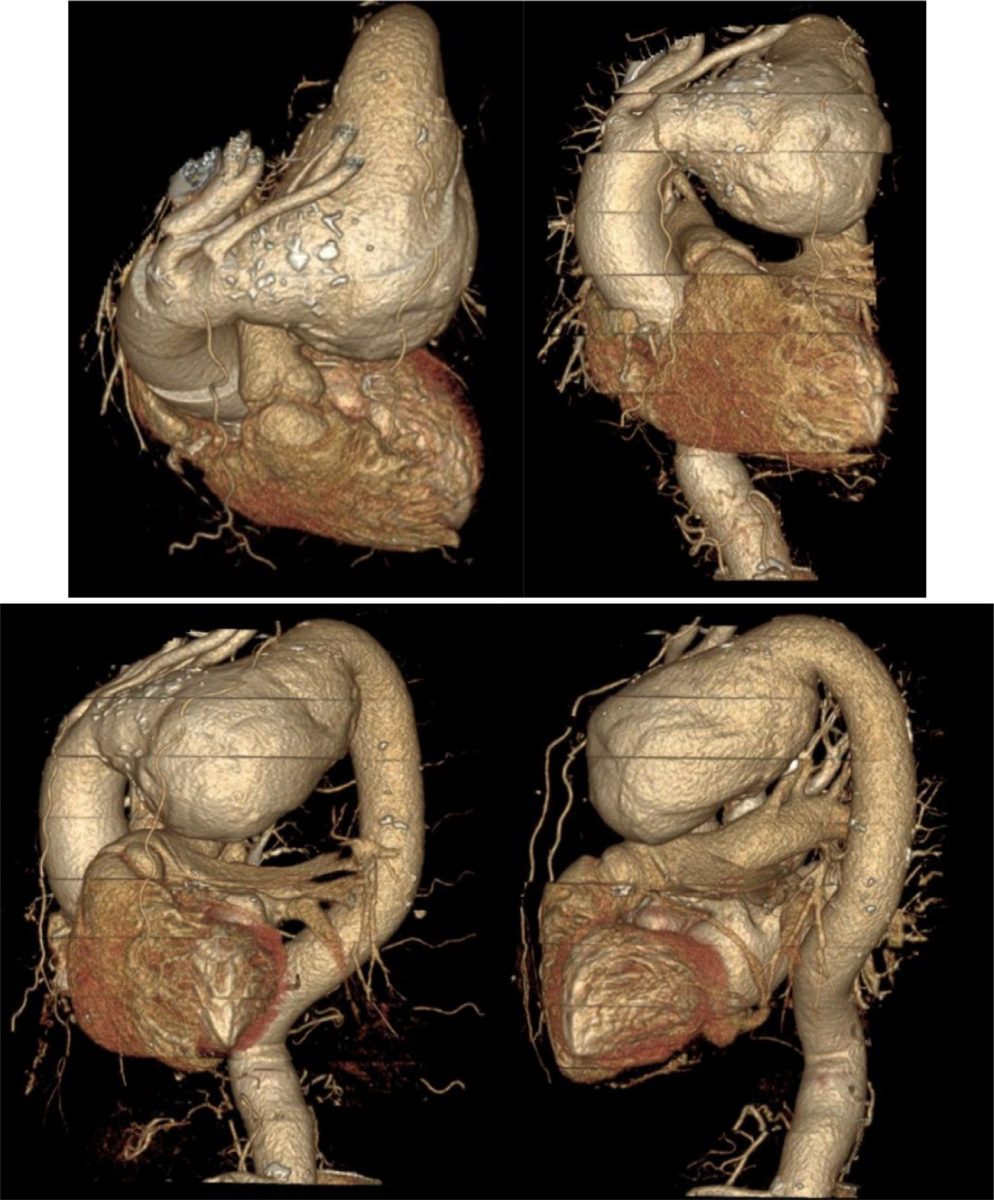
Three-dimensional volume-rendered images of cardiac computed tomography scan showing giant ascending aortic aneurysm.
